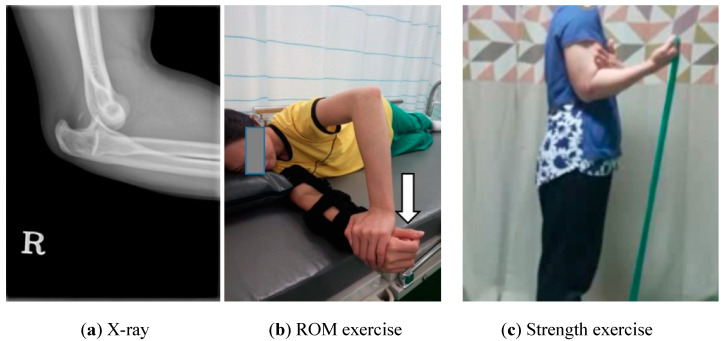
Case A’s X-ray, and range of motion (ROM) and strength exercise.
